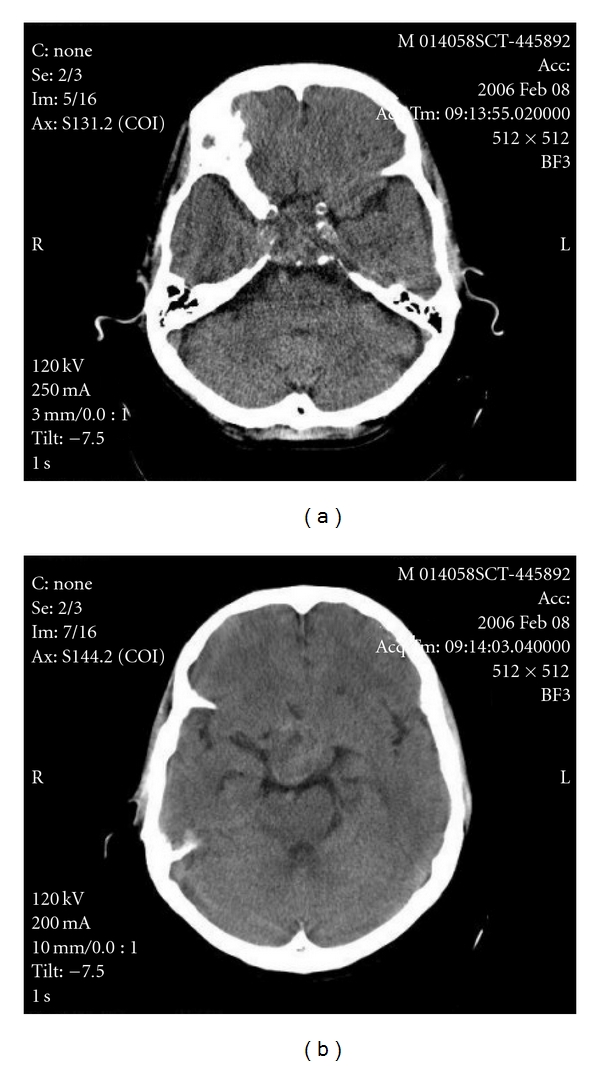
(a) and (b) CT shows a large heterogeneously contrast-enhanced intrasellar mass (3.6 cm in the largest diameter), with suprasellar extension, invasion of the sphenoid sinus, and erosion of the sellar floor.
